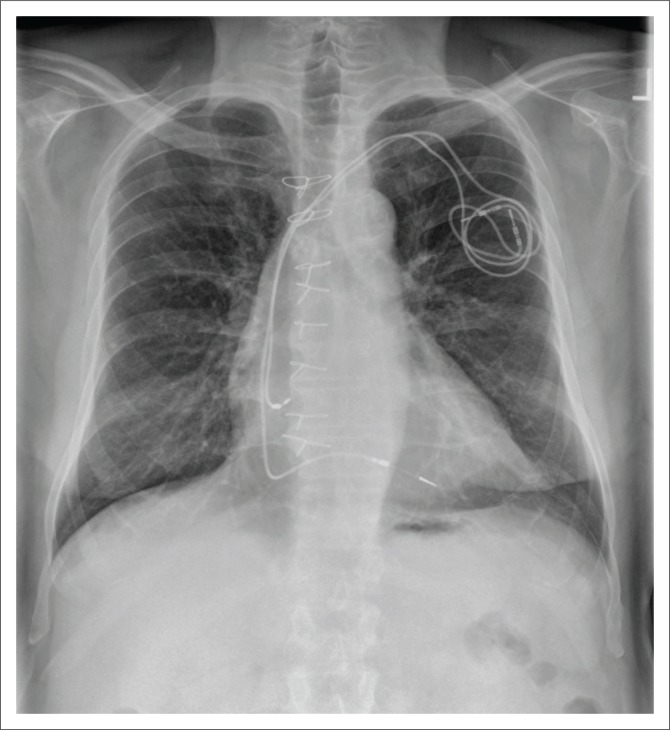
A chest radiograph (CXR) showing abandoned pacemaker leads in the right atrium and the right ventricle.
